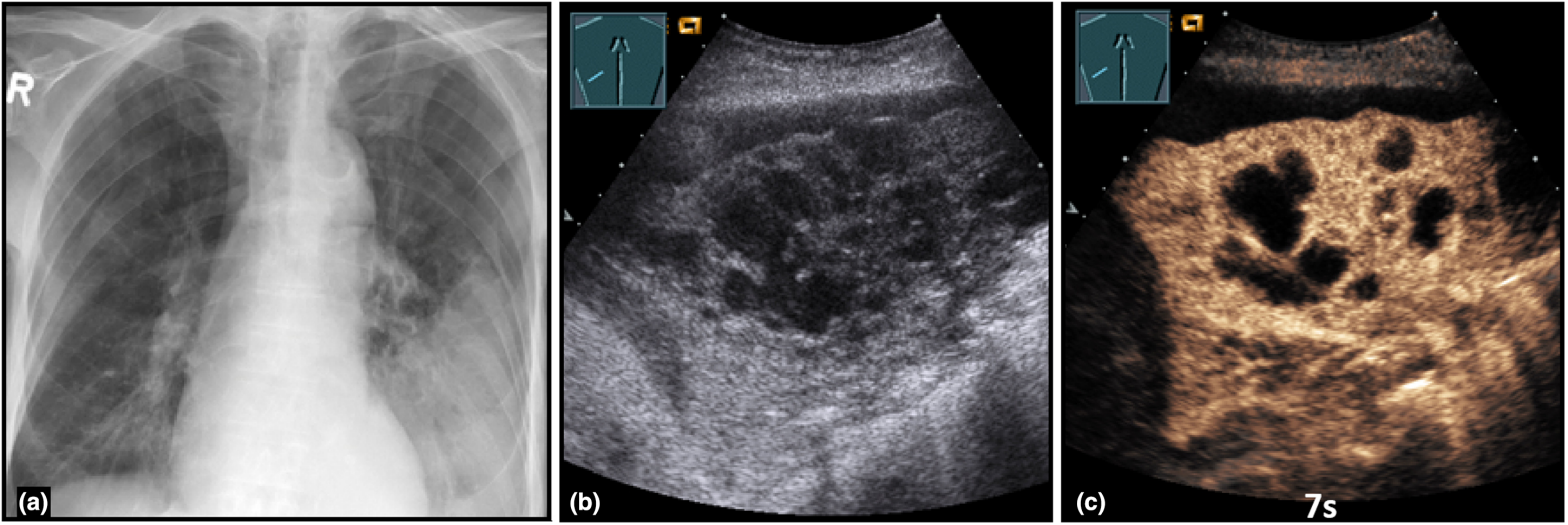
An 84‐year‐old patient with cachexia, general weakness, dry cough, and purulent sputum presented to the emergency department. Presentation of a left‐sided, low‐field consolidation on chest X‐ray (courtesy of Prof. Dr. Andreas H. Mahnken, Department of Radiology, University Hospital Marburg) (a); B‐mode ultrasound (b) shows a complex, hypoechoic consolidation with central, anechoic, confluent areas; contrast‐enhanced ultrasound (c) reveals an inhomogeneous, pulmonary arterial pattern of enhancement after 7 seconds, with multiple anechoic areas with an absence of enhancement, consistent with community‐acquired abscessed pneumonia.
